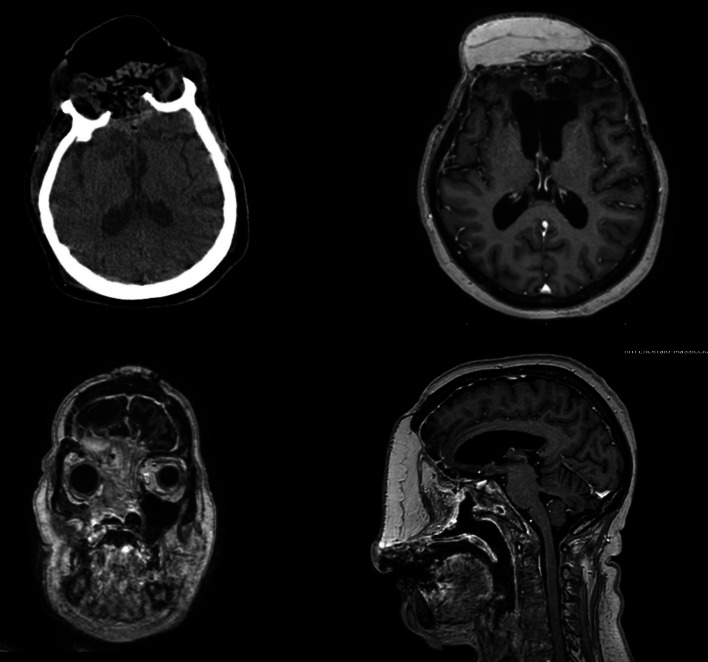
Postoperative MR images in axial, coronal and sagittal views showing the free flap, skin, subcutaneous and muscular layers in the absence of local recurrence of the AMLB
